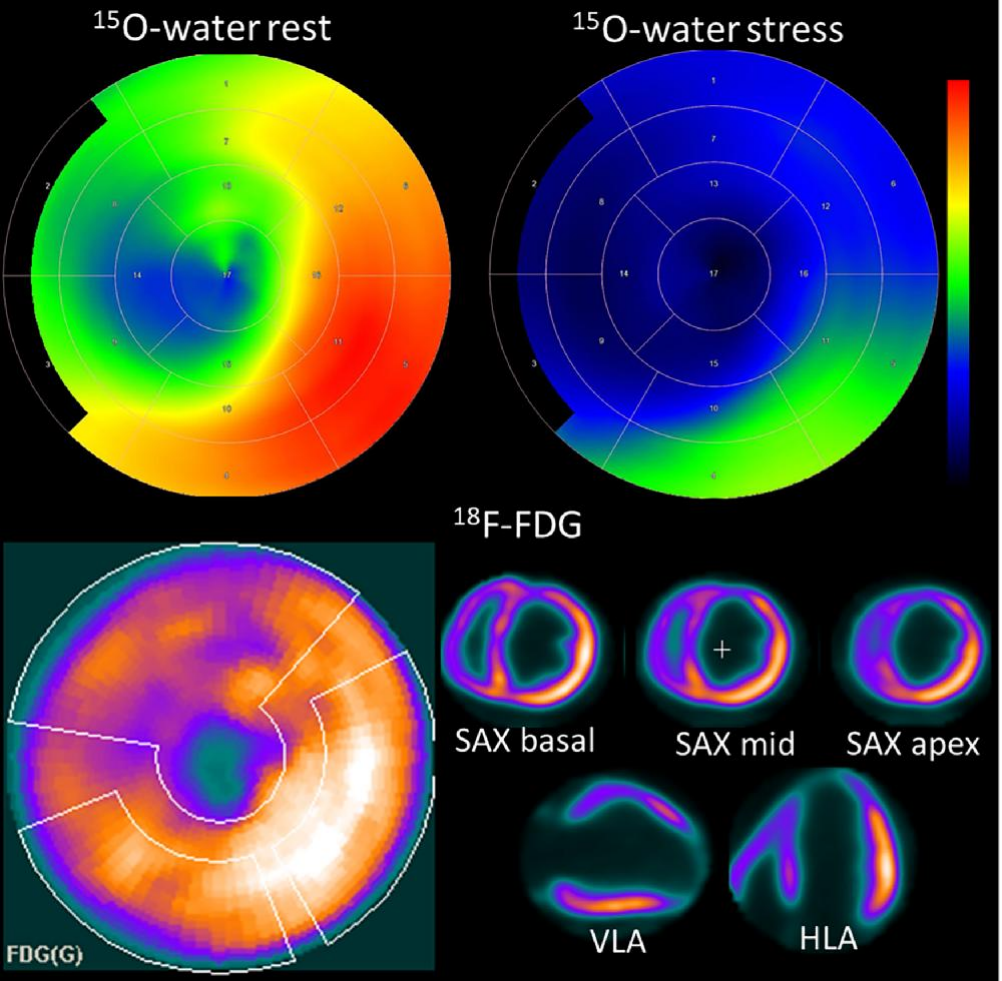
Evaluation of myocardial ischaemia and viability in ischaemic heart failure. The patient had 3-vessel obstructive coronary artery disease, severe wall motion abnormality in the territory of the left anterior descending (LAD) coronary artery, reduced left ventricular ejection fraction (35%), and high surgical risk due to co-morbidities. ^15^O-water PET showed globally reduced stress myocardial blood flow (scale is 0–3.5 mL/g/min). Resting myocardial blood flow (scale 0–1.0 mL/g/min) was also reduced in the LAD territory. ^18^F-FDG PET showed the absence of glucose metabolism in the apex indicating scar but partially preserved glucose metabolism elsewhere in the LAD territory indicating the presence of viable myocardium. The patient underwent successful coronary artery bypass surgery and ejection fraction was improved (45%) at follow-up. HLA, horizontal long axis; SA, short axis; VLA, vertical long axis.
